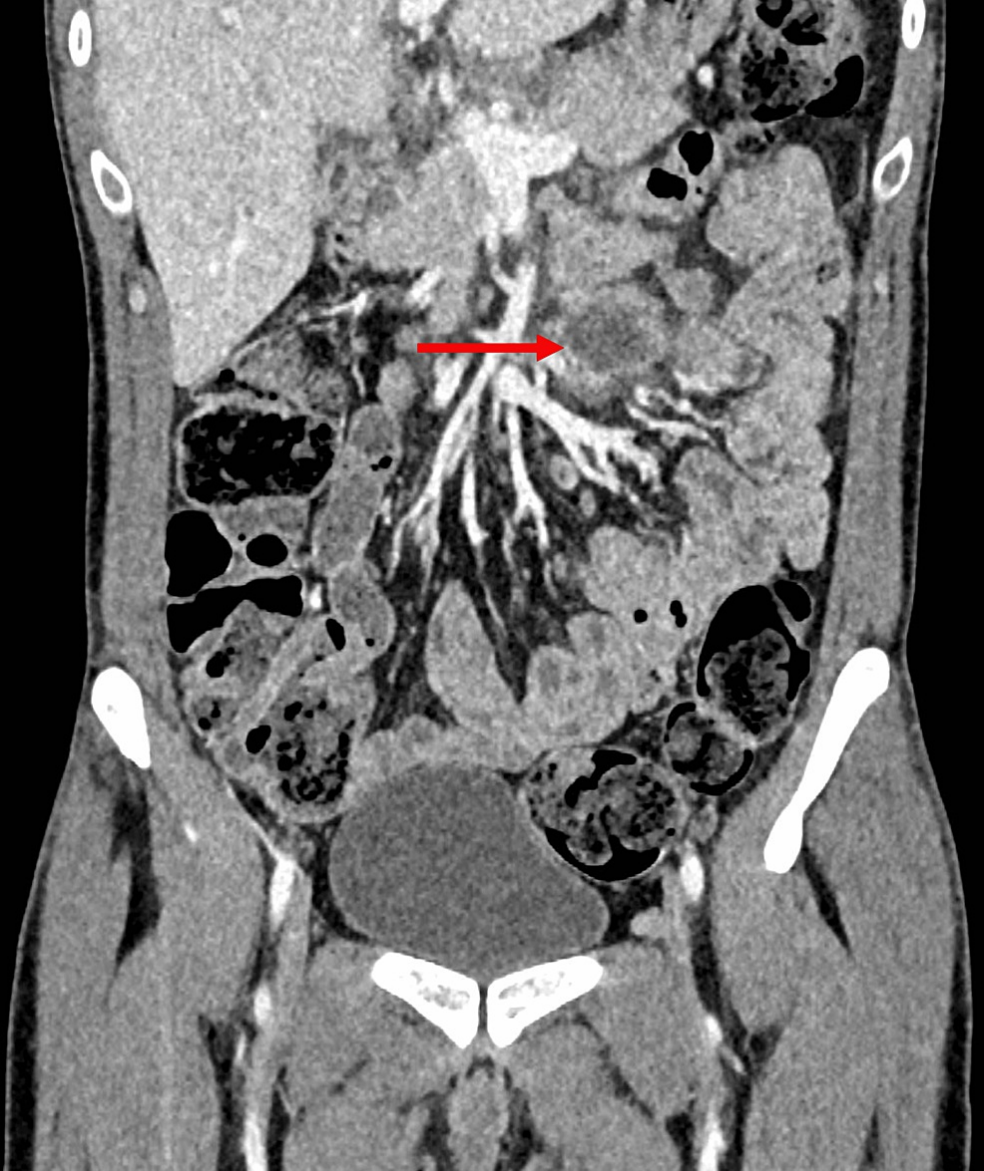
Coronal reformat CT of the abdomen and pelvis A CT of the abdomen and pelvis with intravenous (IV) contrast coronal reformat demonstrating an enlarged conglomerate mesenteric lymph node with internal areas of non-enhancement centered in the left hemiabdomen (red arrow).
